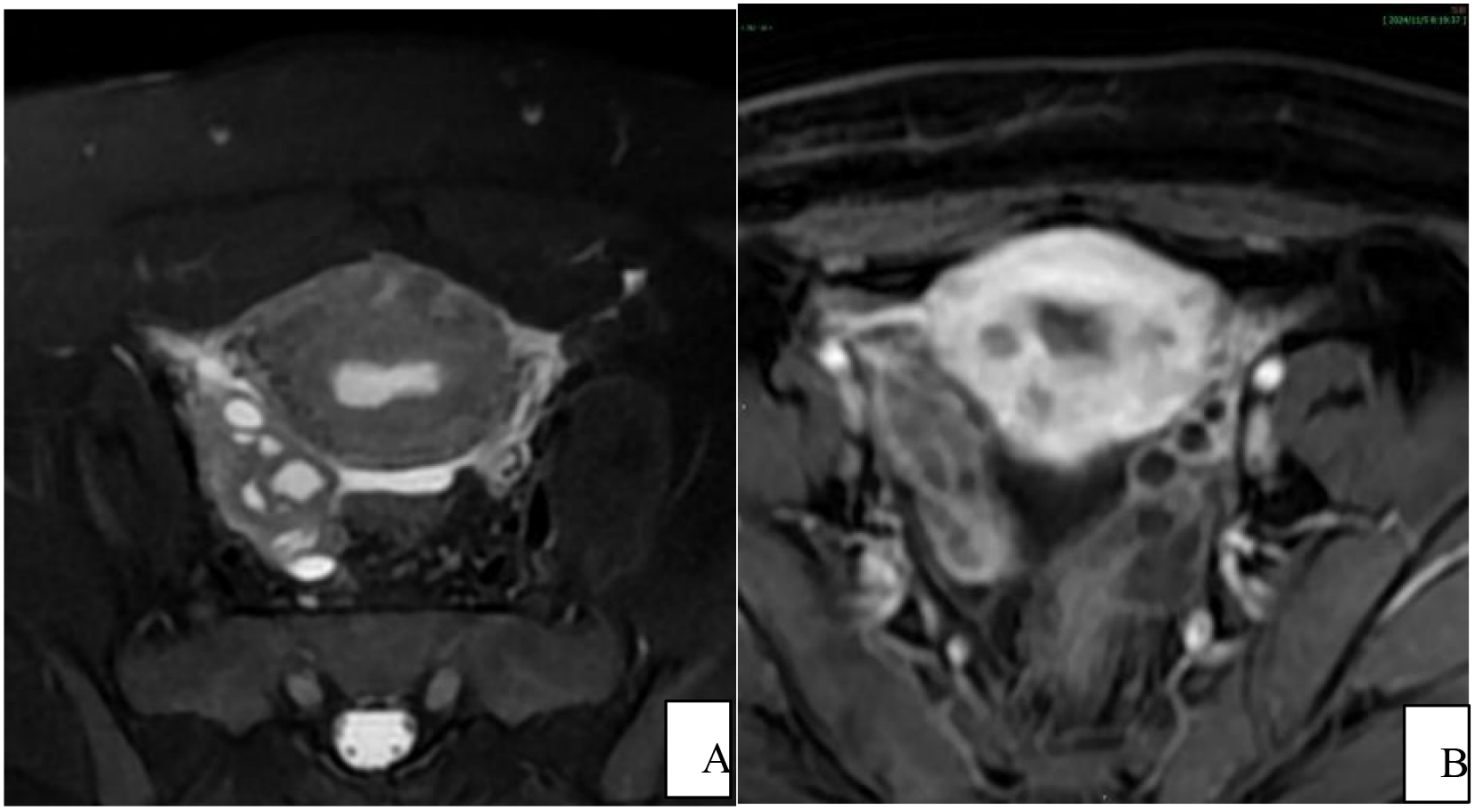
Pelvic MRI axial view. (A) T2WI shows uneven signal intensity in the uterine wall; the right ovary is enlarged, with multiple cystic high-signal intensities inside; (B) In the arterial phase of enhancement, multiple low-enhancement foci are seen in the uterine wall; multiple cystic shadows with wall enhancement are seen in the right ovary.
